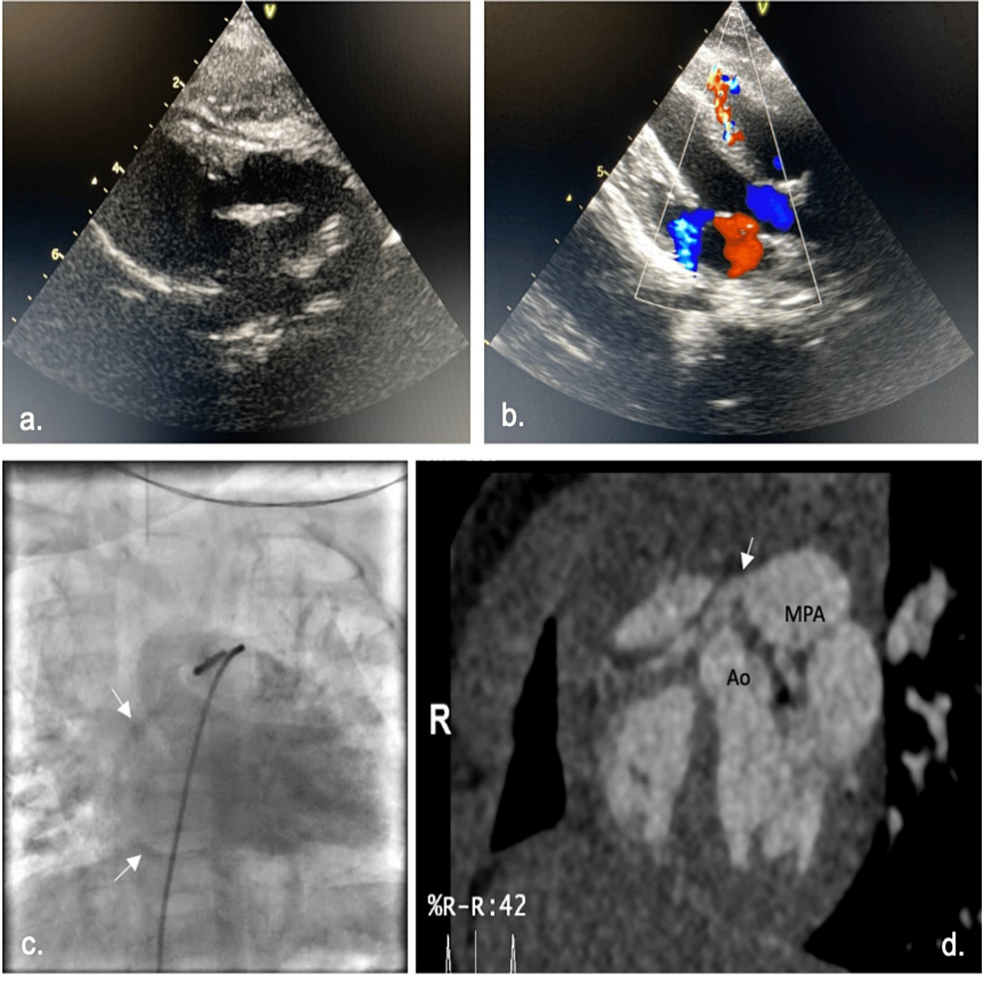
Multimodality imaging workup for the diagnosis of anomalous right coronary artery from the pulmonary artery. (a and b) Echocardiogram demonstrating the course of the RCA above the aorta and color flow Doppler revealing an anomalous coronary branch into the right ventricle (due to collateral circulation) with mitral regurgitation. (c) MPA angiography injection revealing ARCAPA (white arrows). (d) Angio-CT shows RCA (white arrow) arising from the MPA. Ao: aorta; ARCAPA: anomalous origin of the right coronary artery from the pulmonary artery; MPA: main pulmonary artery; RCA: right coronary artery.
